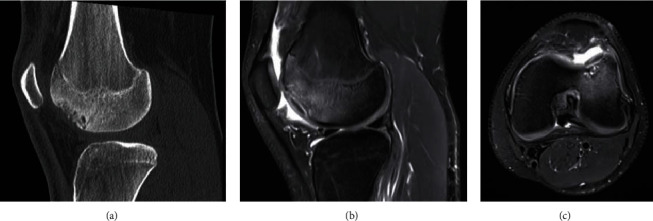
Tomographies of the left knee in a 17-year-old male. (a) CT through the lateral femoral condyle in a sagittal plane showing subchondral cysts. (b, c) MRI in T2-weighted sagittal and axial planes showing subchondral fluid as a sign of dissecate instability.
